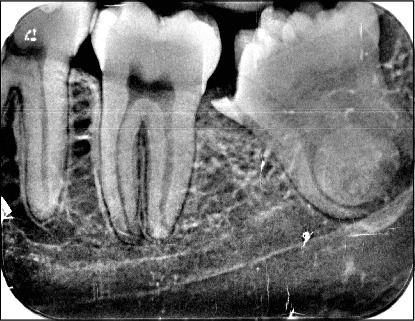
Endoral x-ray shows the absence of enamel and dentin tissue, the relationship between the tooth root and the mandibular canal, and coronal-distal radiolucency.
